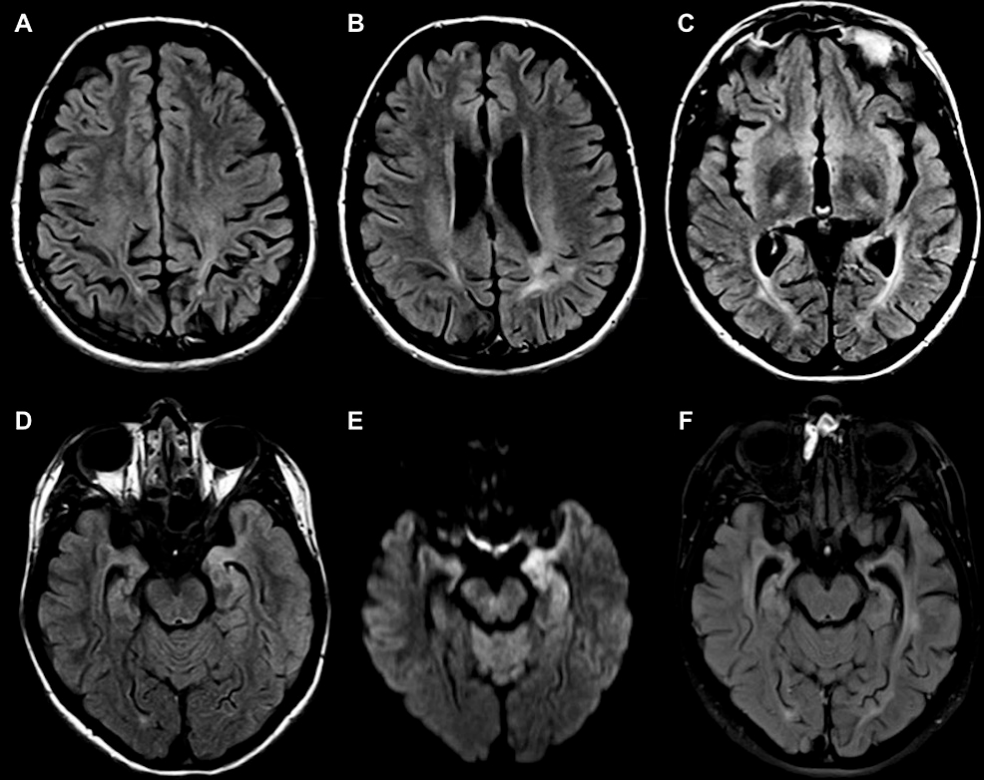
Axial FLAIR images at presentation and two months later Axial FLAIR images at presentation (A-E). There is diffuse brain volume loss out of proportion for age, with ill-defined confluent white matter hyperintensities in bilateral parietal lobes and periatrial regions. The subcortical U-fibers are preserved. There is also asymmetric FLAIR hyperintensity of the left mesial temporal lobe (D) with associated reduced diffusion (E). Axial FLAIR image two months later (F) demonstrate the progression of left mesial temporal atrophy, with a resolution of reduced diffusion (not shown). FLAIR: fluid-attenuated inversion recovery.
